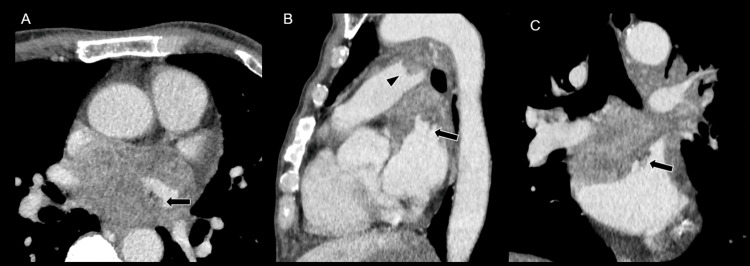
Chest CT. Chest contrast-enhanced CT demonstrates left atrial invasion (A-C, arrows), and pulmonary artery invasion (B, arrowhead) of the mediastinal metastatic tumor.
